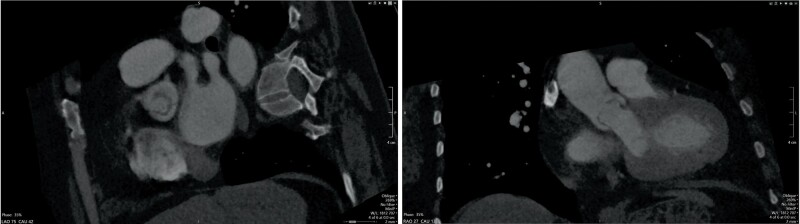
CT TAVR (35% phase) shows aortic valve opening appears eccentric, loophole-shaped on the short axis view (left); systolic dooming of the aortic valve leaflets on the long axis view (right).
